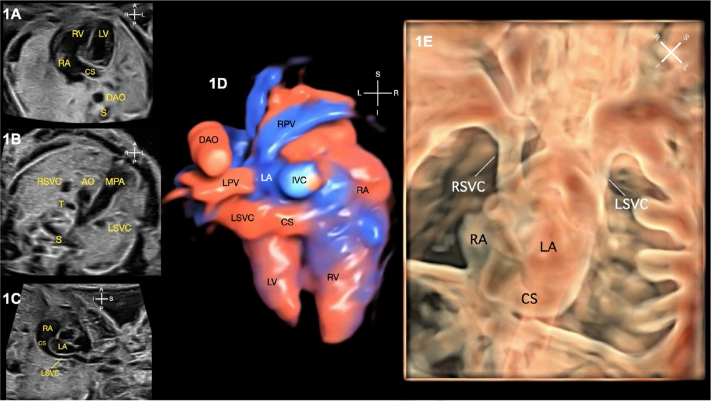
2-and 3-Dimensional Fetal Echocardiography of Bilateral Superior Vena Cava in a 24 Weeks Fetus (A to E) Bilateral SVC. (A) Four-chamber view showing dilated CS. (B) Four vessels in 3-vessel view. (C) Gray-scale image showing the entire LSVC drainage into the CS in sagittal view. (D and E) Four-dimensional STIC power Doppler (D) and HD live tissue surface rendering (E) images showing LSVC draining through CS into RA. AO = ascending aorta; CA = celiac axis; CS = coronary sinus; DAO = descending aorta; IVC = inferior vena cava; LA = left atrium; LPV = left pulmonary vein; LSVC = left superior vena cava; LV = left ventricle; MPA/PA = main pulmonary artery; RA = right atrium; RPV = right pulmonary vein; RSVC = right superior vena cava; RV = right ventricle; S = spine; STIC = spatiotemporal image correlation; SVC = superior vena cava; T = trachea.
